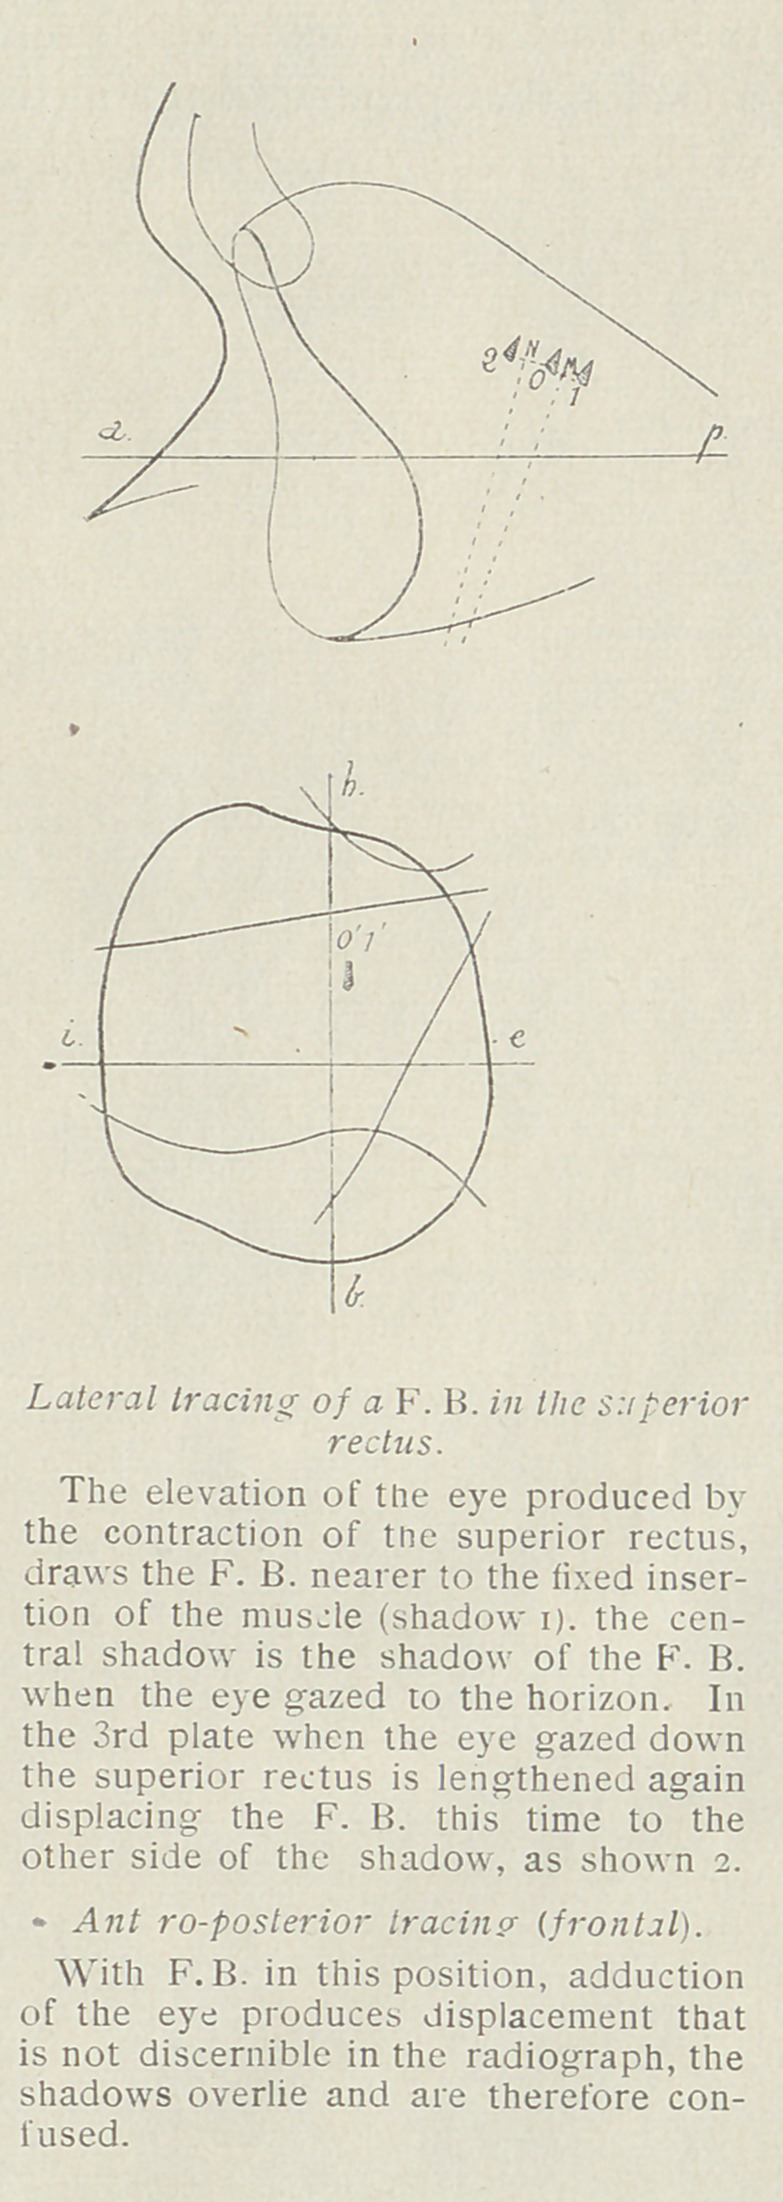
# X-Ray Examination for Foreign Bodies in the Eye and Their Localization
